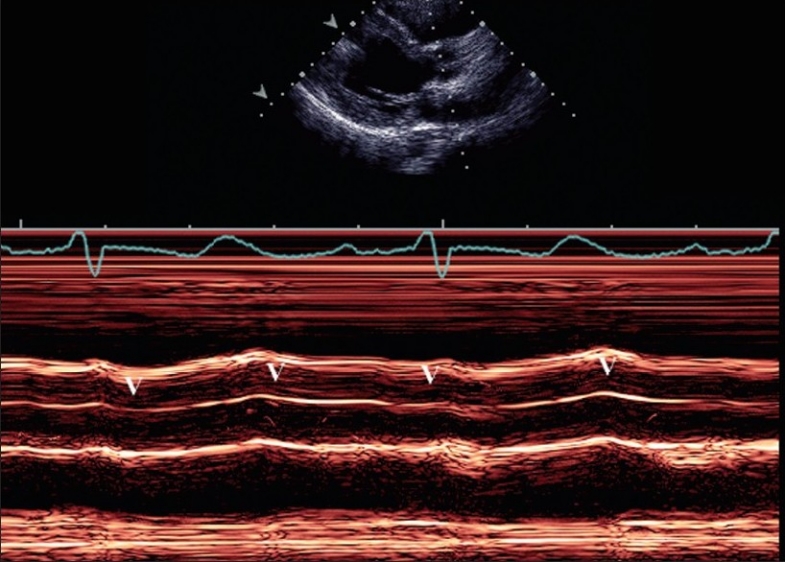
Post-LVAD implantation M mode echocardiogram of the aortic valve and left atrium shows closed aortic cusps throughout systole and diastole (v), denoting the well-functioning LVAD
